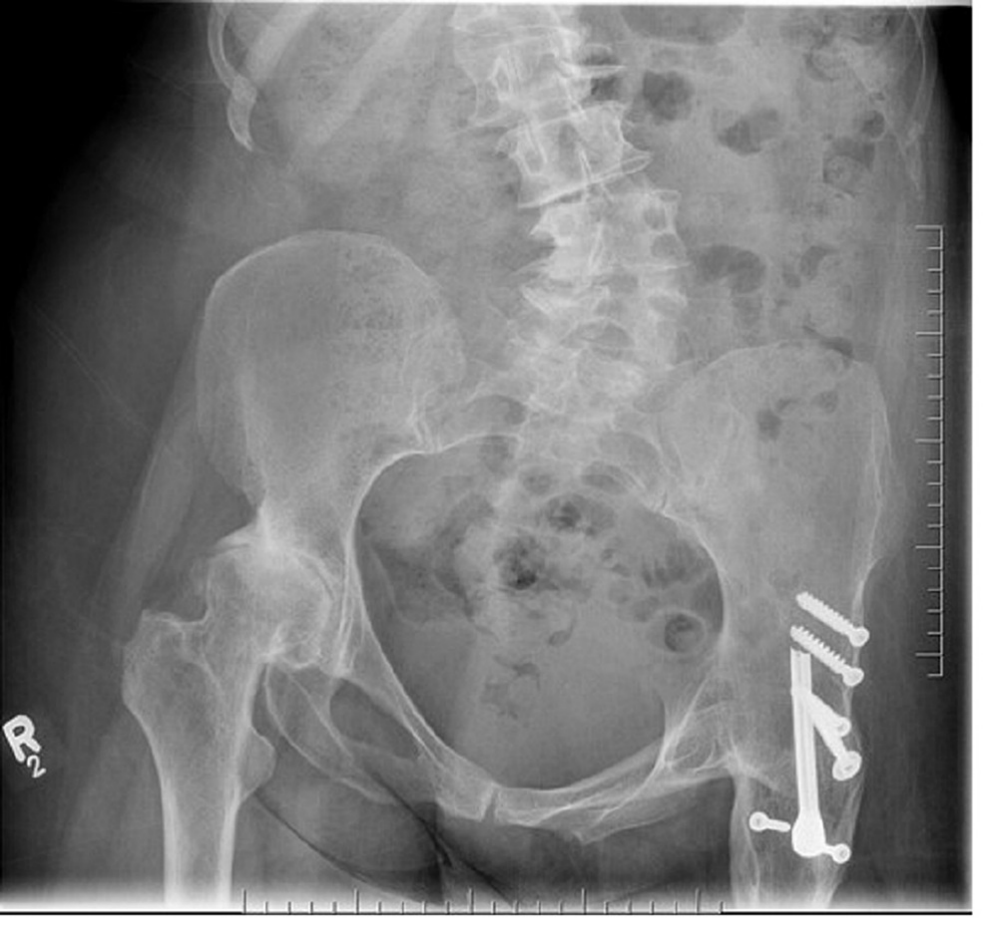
Pre-operative image Pre-operative anteroposterior radiograph of the pelvis, depicting left-sided hip arthrodesis. Advanced degenerative scoliosis of the lumbar spine is also noted.
